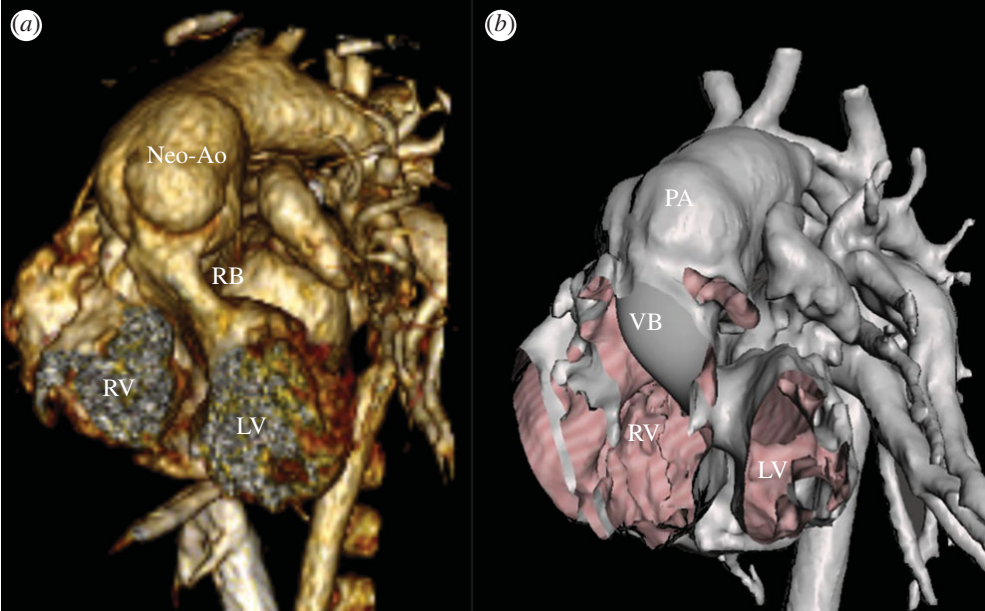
Comparison of geometries following surgical biventricular repair of DORV case Pt11: (*a*) post-procedural CT reconstruction highlighting right ventricle (RV), left ventricle (LV), neo-aorta (Neo-Ao) and real baffle (RB); and (*b*) virtual geometry including RV, LV, pulmonary artery (PA) and virtual baffle (VB). The PA was not switched to Neo-Ao in the model. (Online version in colour.)
